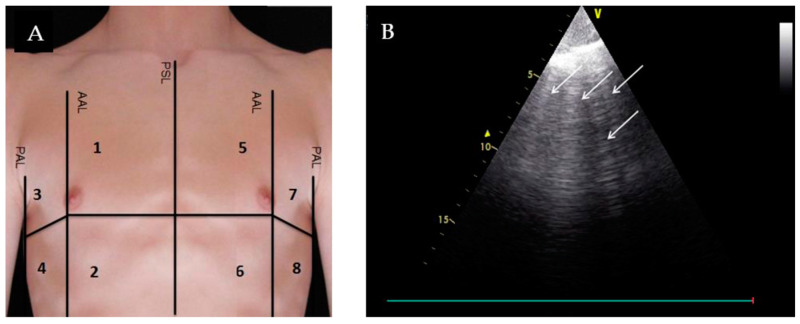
Detection of B-lines by lung ultrasound. (A) Division of the thorax in eight lung zones. PSL: parasternal line; AAL: anterior axillary line; PAL: posterior axillary line; (B) Example of a lung ultrasound loop of quadrant 1 at admission assessed as a positive region due to the appearance of ≥3 B-lines. Reproduced with permission from Glöckner et al., *Medicina*
